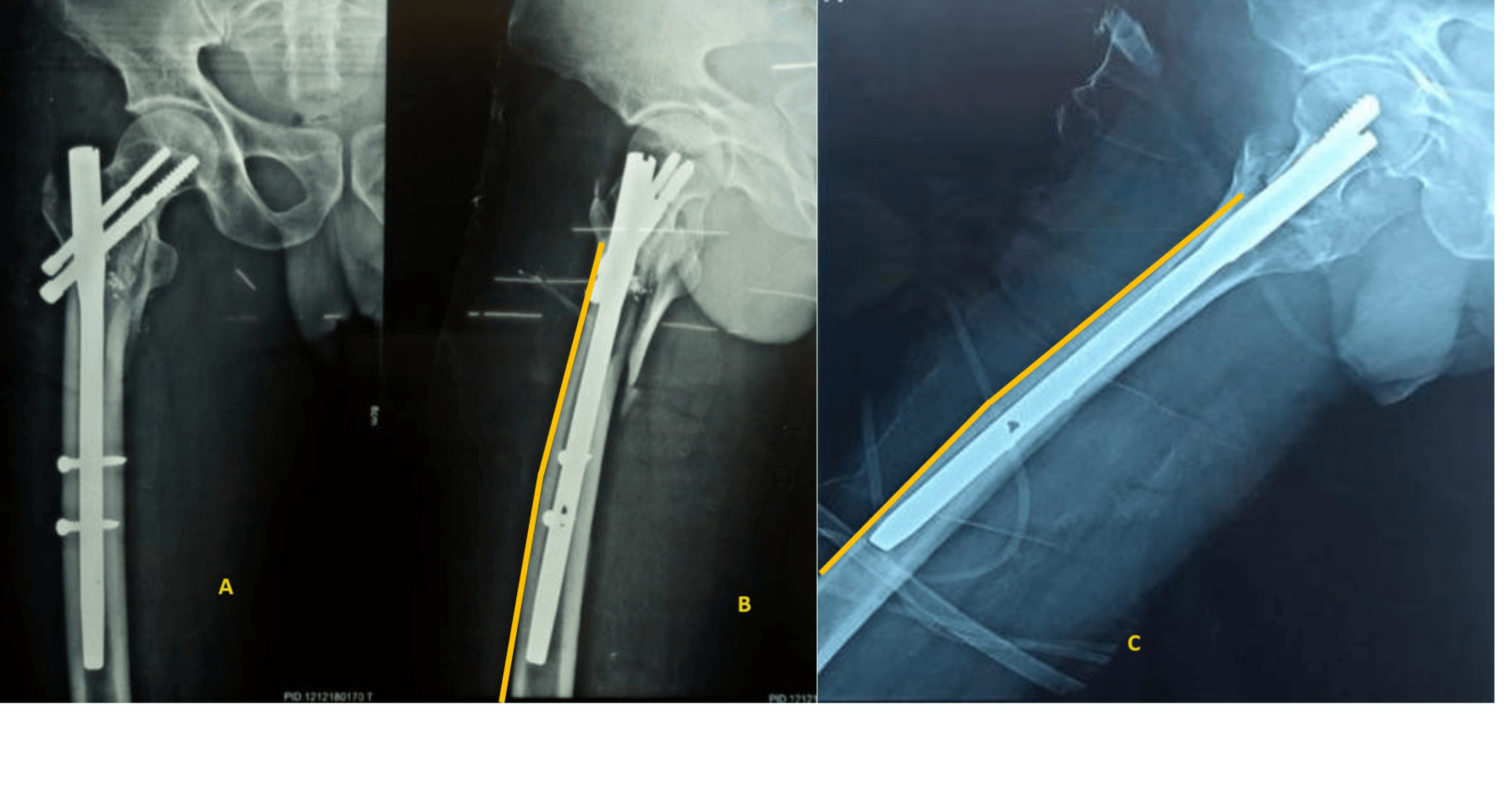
X-ray of hip with proximal femur showing anterior cortex (AI cortex) (A) Antero posterior view; (B) and (C) lateral view; yellow line: tangential line parallel to anterior cortex of femur proximal and distal to the most bowed point of the femur
